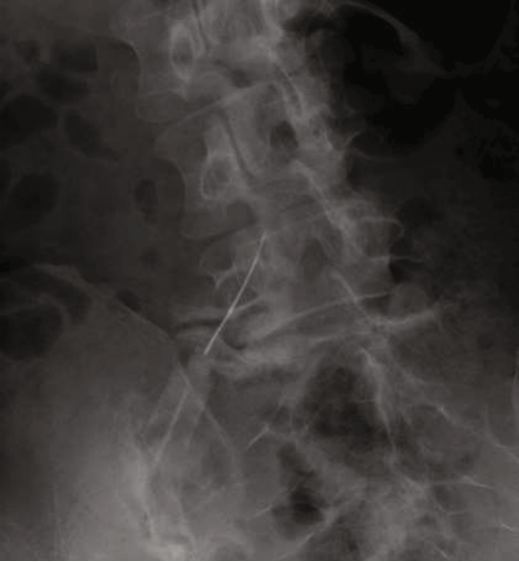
X-ray during left L4/5 facet puncture.
